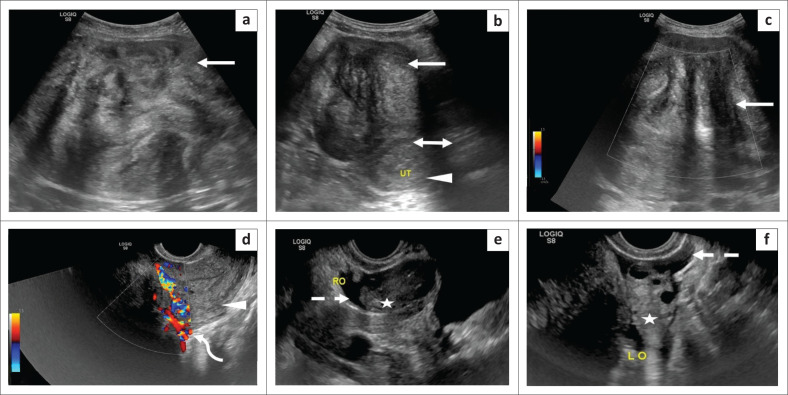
Grey-scale and Doppler ultrasound. Transverse (a) and sagittal (b) grey scale transabdominal ultrasound images of the abdomen and pelvis depict a large, well-circumscribed, heterogeneous, solid abdomino-pelvic mass (arrow), abutting the uterine fundus (double arrow in b) with no internal colour flow on Doppler evaluation (c). Transvaginal sagittal Doppler ultrasound image (d) demonstrates the vascular pedicle (curved arrow) between the uterus (arrowhead) and the mass, suggesting its uterine origin. Both ovaries were normal (⋆) and visualised separate from the mass with a small amount of anechoic free fluid in pelvis (dashed arrow) on transvaginal transverse (e) and sagittal grey scale images (f).
